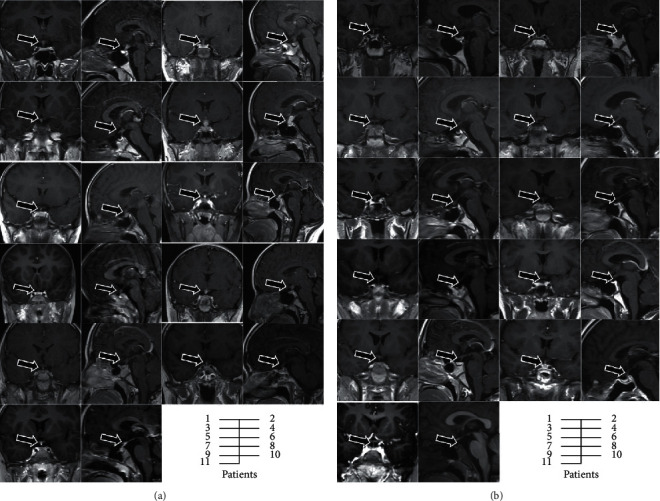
Contrast-enhanced brain magnetic resonance imaging of patients: (a) coronal/sagittal-enhanced brain magnetic resonance imaging at diagnosis and (b) coronal/sagittal-enhanced brain magnetic resonance imaging after chemoradiotherapy (tumor is marked with the arrow).
